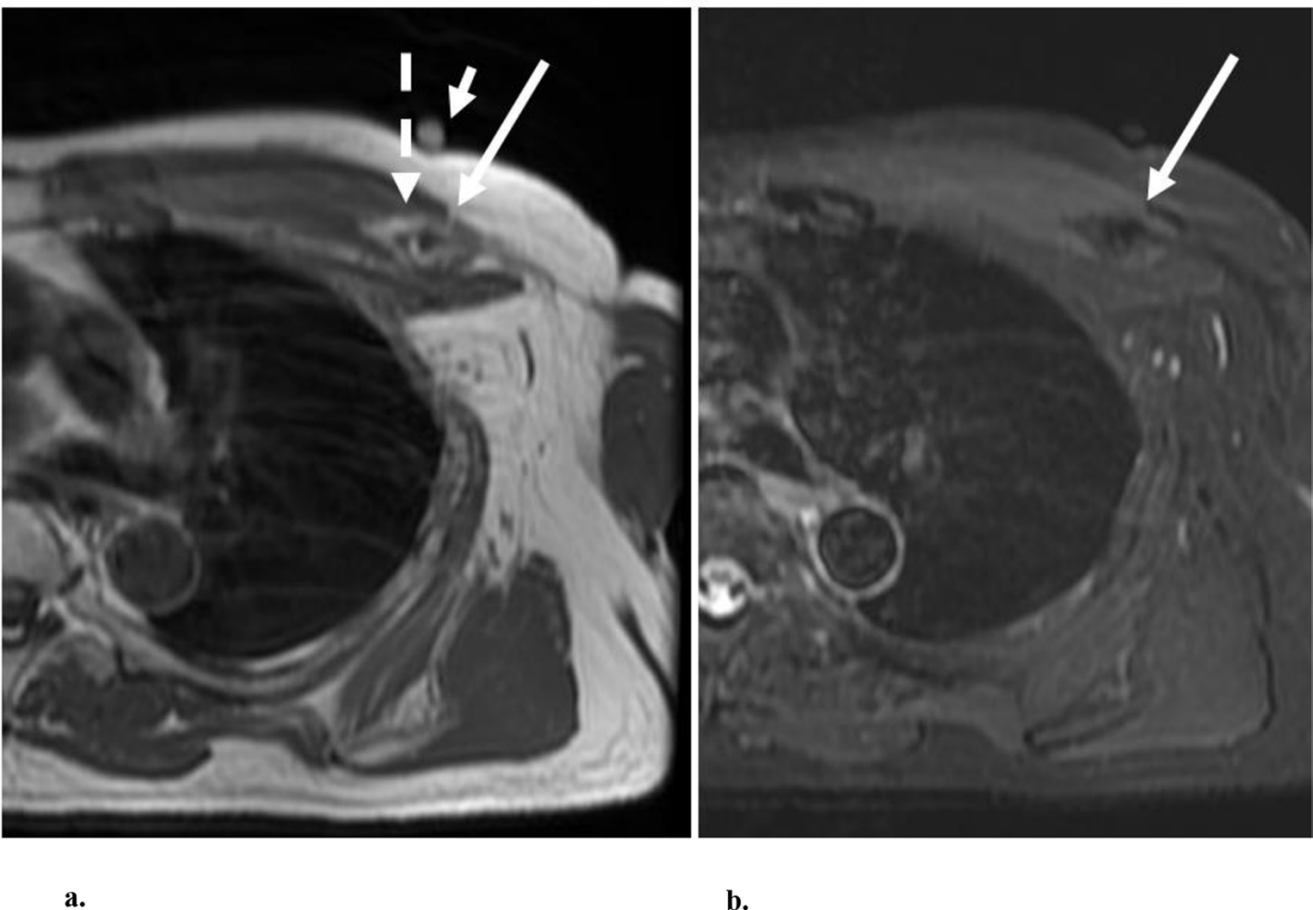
45-year-old-male that presented with left anterolateral chest pain underlying the marker (short arrow in a) approximately 8 months after injury of the left pectoralis major from assault. (a) Axial T1-weighted MR image shows nodular signal, which is relatively hypointense to muscle, in the region of the left pectoralis major myotendinous junction (long arrow). Note surrounding fat signal (dashed arrow). (b) Axial STIR-weighted MR image shows the corresponding low signal at this site (arrow) and the absence of edema. At surgery, there was scar tissue and a complete tear at the myotendinous junction of the sternal head (full-width partial-thickness) of the pectoralis major
